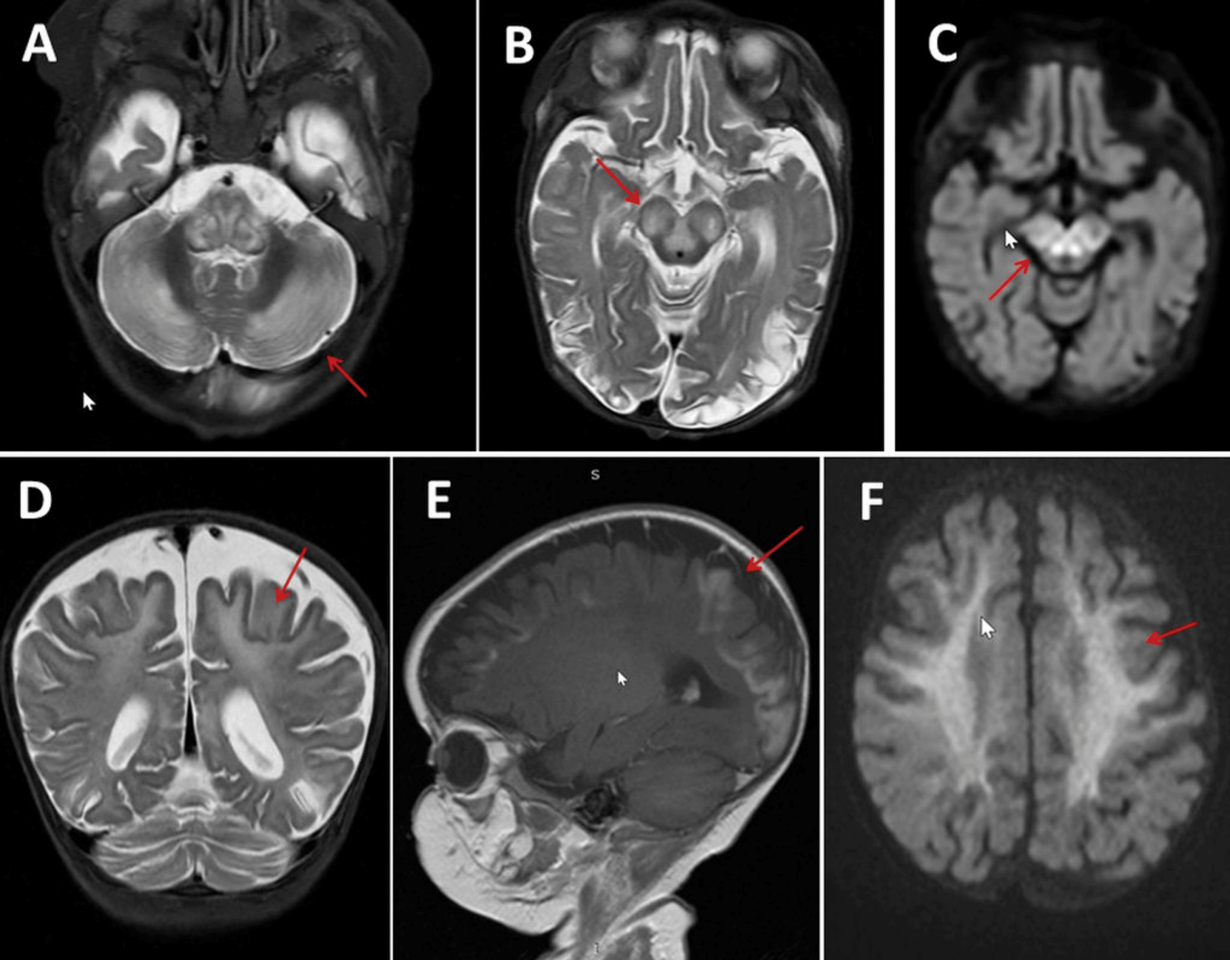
Cerebral magnetic resonance imaging showing symmetrical T2-hyperintense lesions in the cerebellar hemispheres (panel A), symmetric T2-hyperintense lesions in the crura cerebri (panel B), symmetric diffusion-weighted imaging (DWI) -hyperintense lesions in the midbrain (panel C), symmetrical deep and subcortical white matter lesions (panel D), T1-hyperintense lesions in the occipital lobe (panel E), and symmetrical diffuse DWI-hyperintensities in the white matter (panel F)
